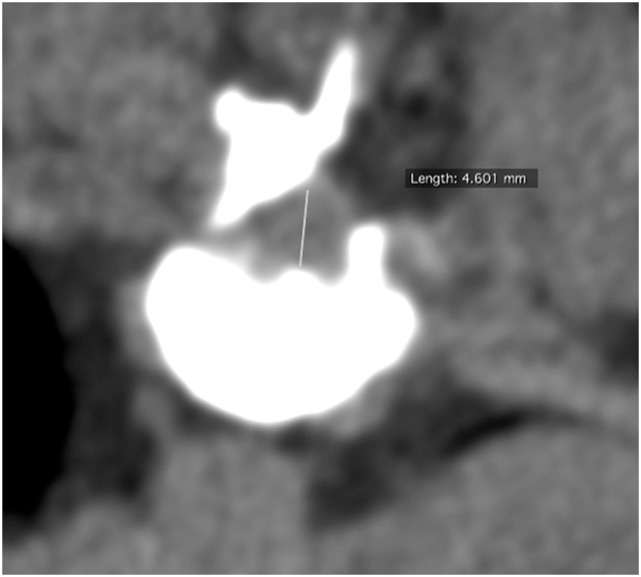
Postoperative (hemilaminectomy) transverse computed tomography image at site of maximal compression with measurement of minimal cross-sectional dimension.
